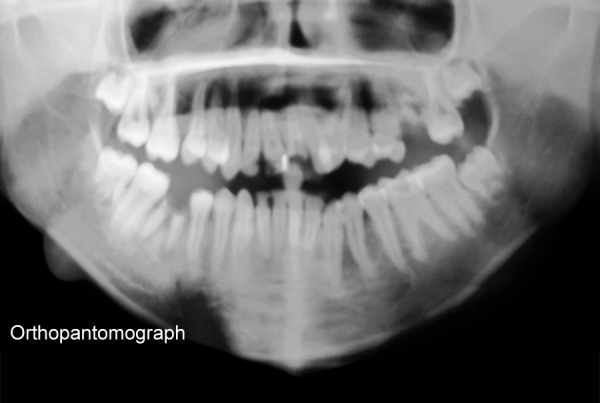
Orthopantomograph showing massive resorption in lower border of mandible
